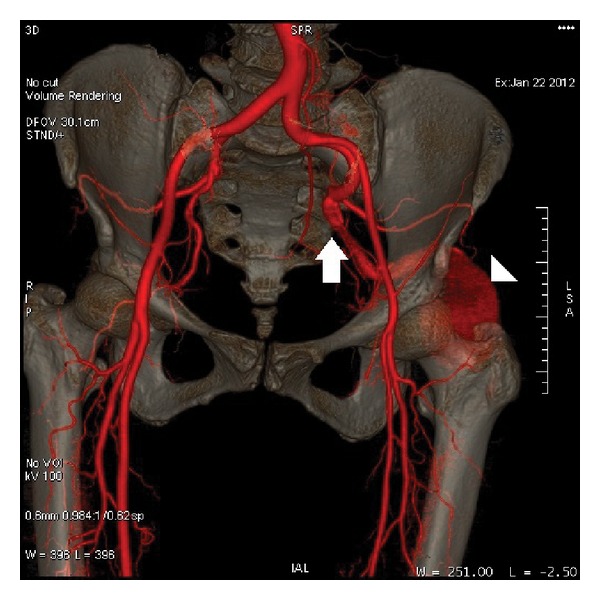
CT angiogram. The arrow depicts the abnormally large internal iliac artery. The arrowhead depicts the aneurysmal dilatation. Note the normal-sized internal iliac artery of the opposite side.
